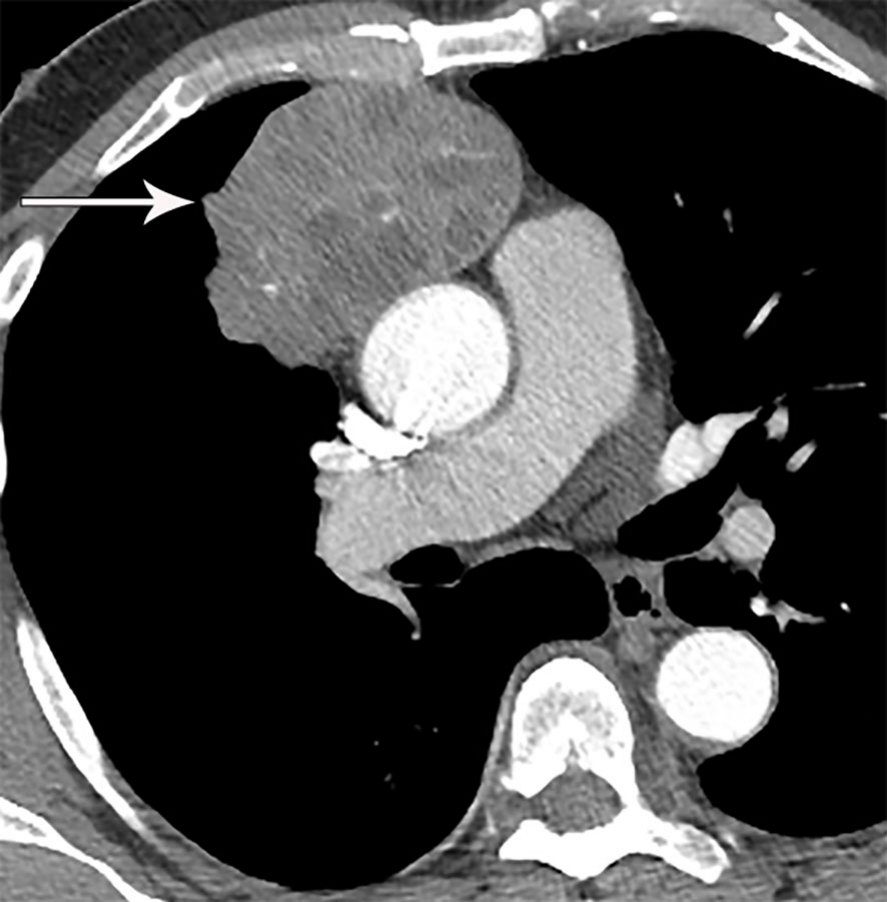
70 year old man with WHO type B3 thymoma. Contrast-enhanced CT shows right prevascular mediastinal mass with heterogeneous attenuation and areas of necrosis (arrow), consistent with more aggressive WHO subtype identified pathologically.
